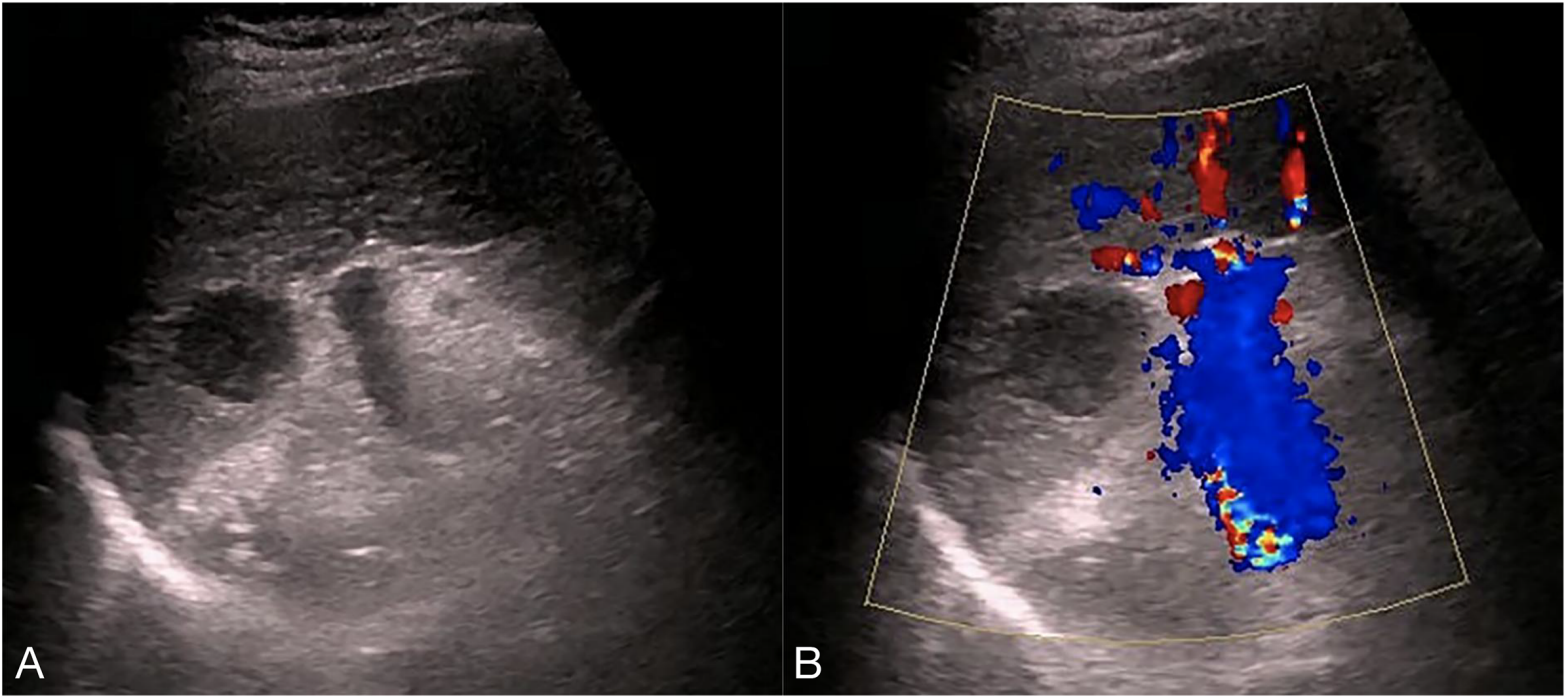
Two-dimensional ultrasound and Color Doppler flow images. (A) the spleen was enlarged, with approximately 52 mm thickness and 147 mm length, diffuse thickening and uneven distribution,of the parenchymal echo, and zonal- or grid-shaped. A mass was observed at the splenic hilum, with a size of approximately 24 × 22 mm, uneven surface, very low internal echo, oval shape, and clear boundary. (B) The mass at the splenic hilum showed a weak blood flow signal, with no apparent abnormality in the blood flow signal of the spleen.
